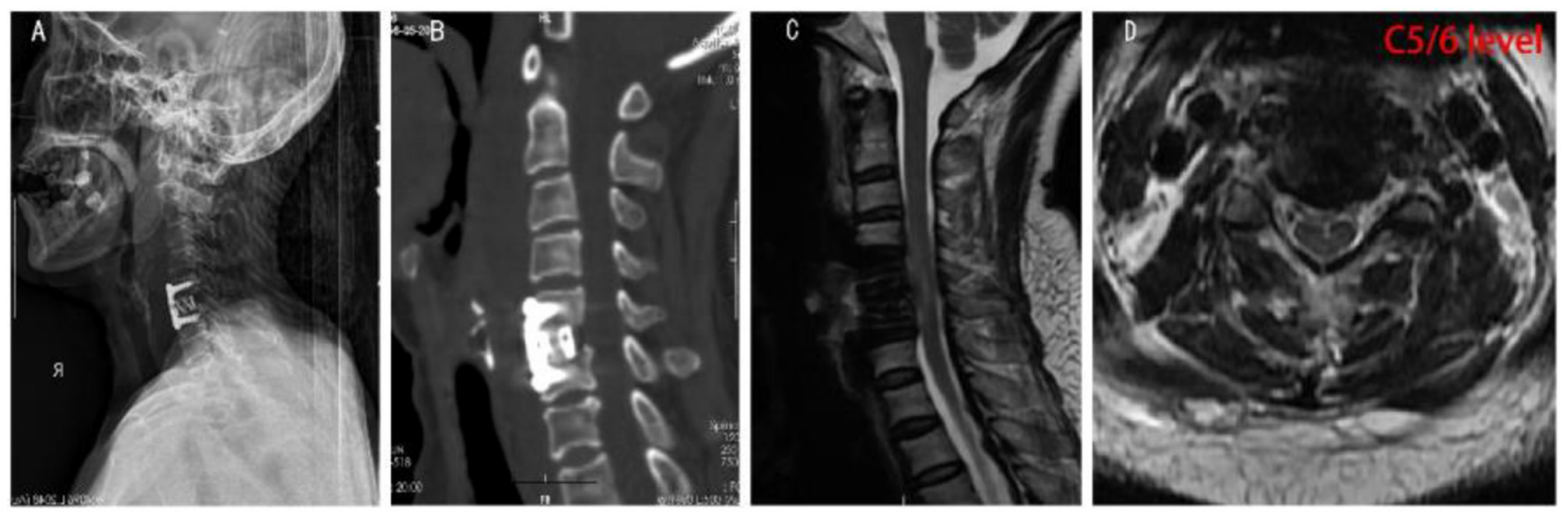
Postoperative imaging results of the patient. (A,B) Postoperative X-ray and CT of the cervical spine showed changes in the C5-6 vertebral body after screw internal fixation, no slippage and fracture were observed, and cage shadow was observed in the C5/6 intervertebral space. (C,D) Postoperative sagittal and transverse MRI of the cervical spine showed internal fixation shadow in the C5-6 vertebrae, showing postoperative changes, with internal fixation in place. No obvious effusion was observed in the C2-T2 prevertebral space.
